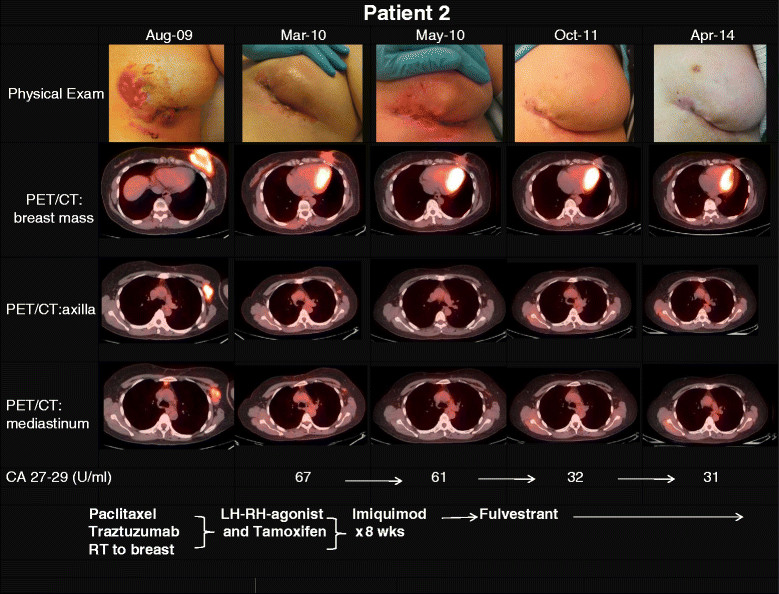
Clinical exam photographs, imaging (PET/CT), tumor marker (CA 27-29) and treatment regimens according to timeline for patient 2. At initial presentation of the patient in August 2009, the locally advanced breast cancer involved the overlying skin, axillary lymph nodes and invaded the pectoralis muscle. In addition an adrenal metastasis and mediastinal lymph nodes were detected by imaging. Interval treatments included paclitaxel, trastuzumab, LH-RH agonist, tamoxifen, topical imiquimod followed by fulvestrant, on which the patient experienced a complete response including breast, lymph nodes and adrenal lesions in October of 2011. At last follow up May 2014, patient had no clinical or radiographic evidence of disease.
